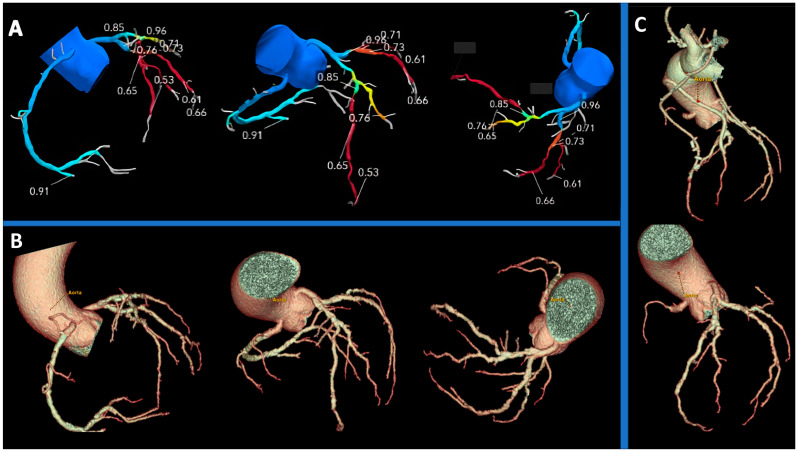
Anatomical evaluation and functional assessment of coronary disease derived from CCTA in a pre-surgical setting. (A) FFR-CT 3D model of the coronary tree, with FFR_CT_ values reported: the left anterior descending and circumflex coronary artery exhibit pathological FFR values (<0.8), while the first diagonal branch demonstrates values close to pathological thresholds. (B) 3D volume rendering reconstruction of the coronary tree provides an assessment of the anatomy in a pre-surgical scenario. (C) 3D volume rendering reconstructions are used to compare graft points decided preoperatively based on FFR values, with post-CABG (upper image) and pre-CABG (lower image) representations. CCTA: Coronary Computed Tomography Angiography; FFRCT: Fractional Flow Reserve Coronary Tomography; CABG: Coronary Artery Bypass Graft.
